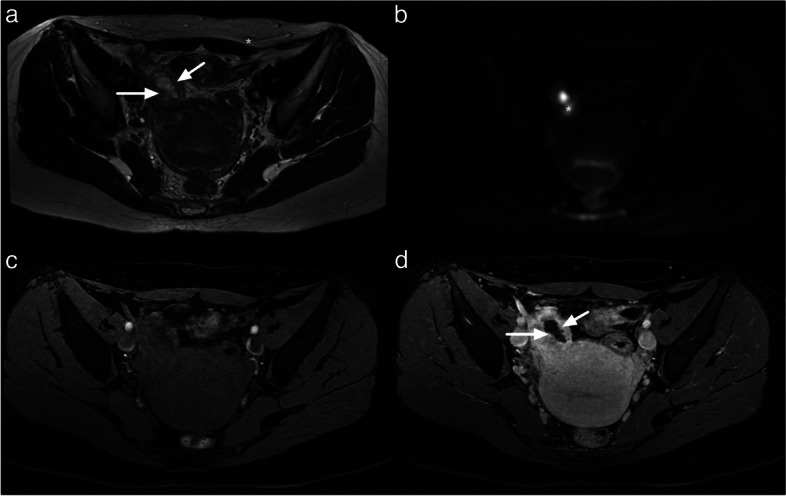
Example of MRI appearance endosalpingial folds. a Axial T2-weighted sequence. b Axial DWI lombo-pelvic scan (*b*: 1200 s/mm^2^). c Axial unenhanced T1-weighted water sequence. d Axial late-gadolinium T1-weighted sequence. Multiple endosalpingial folds (white arrow) potentially leading to a misinterpretation with ovarian vegetations. Note the hypersignal on the DWI scan corresponding to pus inside the fallopian tube (white star)Fimbriated end of the tube may look like irregular solid tissue with strong enhancement and may be recognized thanks to its stellar morphology (Fig. 12)
